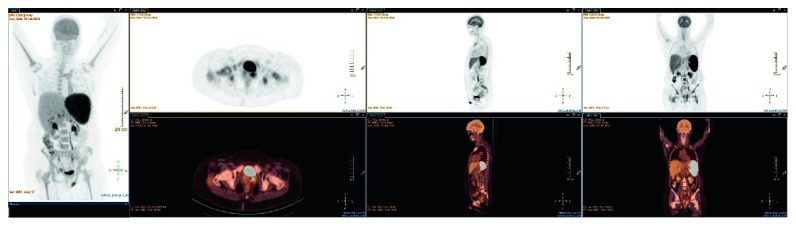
PET-CT hypermetabolic splenomegaly, perihepatic hypermetabolic lymph nodes, and cervical hypermetabolism.
